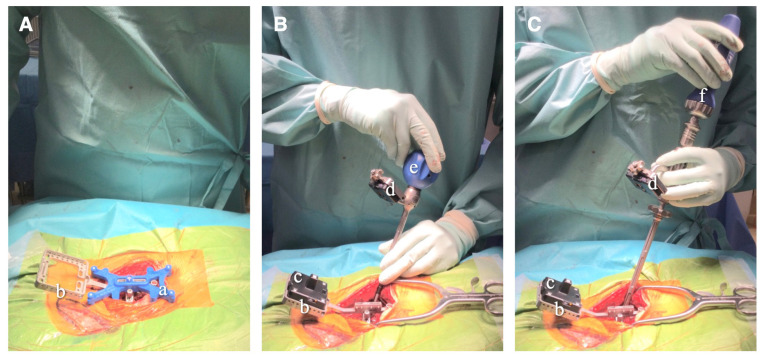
Surgical procedure for pedicle screw insertion under NextAR navigation. (A) During the intraoperative CT scan, the fiducial block (a) is placed over the clamp (b), which is attached to the spinous process. (B,C) The NextAR tracking system includes the target (c) mounted to the clamp (b) and the infrared camera (d) mounted on surgical instruments, such as the pedicle probe (e) and pedicle screwdriver (f).
